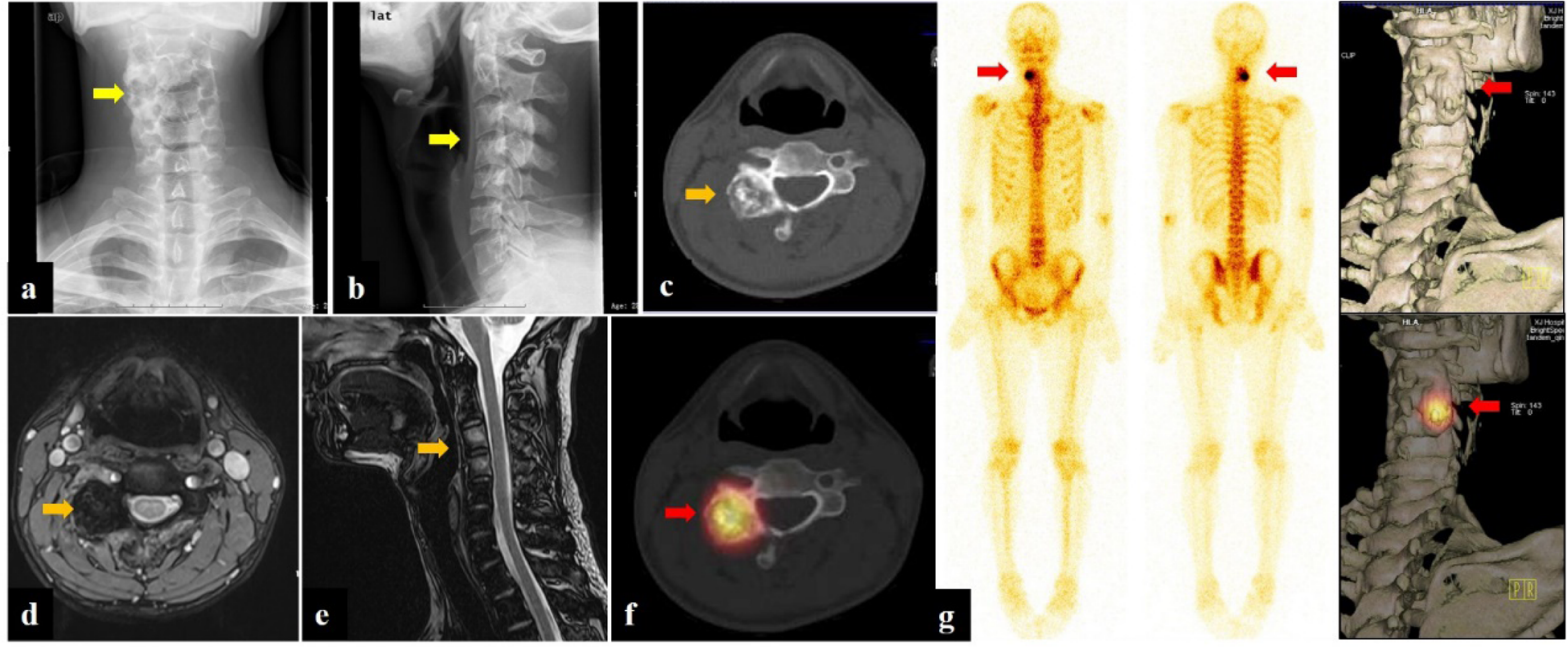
Patient no. 10, male, 16 years old. Planar bone scan (a) demonstrated high uptake in the right attachment of the L4 level, indicating strong osteogenesis. SPECT/CT imaging (b) and 3D reconstruction images (c, d) clearly showed the center solid nidus with peripheral osteosclerosis
